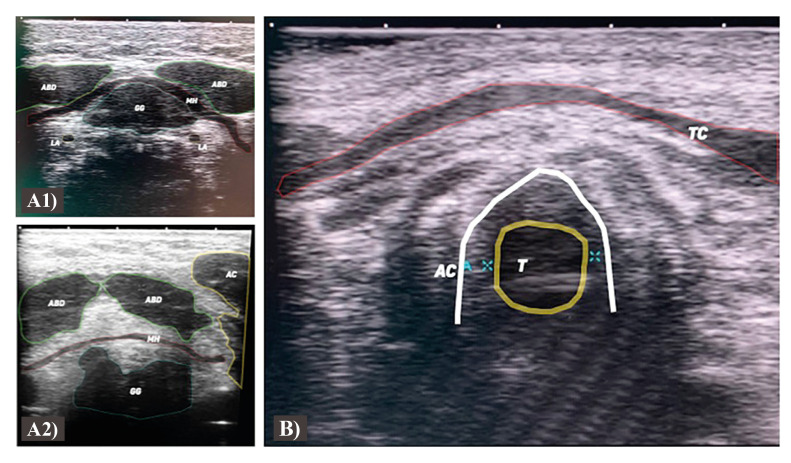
A) Ultrasonography image of the sublingual and mylohyoid region revealing the anterior belly of the digastric muscle, mylohyoid muscle, central raphe, genioglossus muscle, and lingual arteries. B) Ultrasonography image of the central cervical area revealing the thyroid cartilage, airway column, and tube placement.
